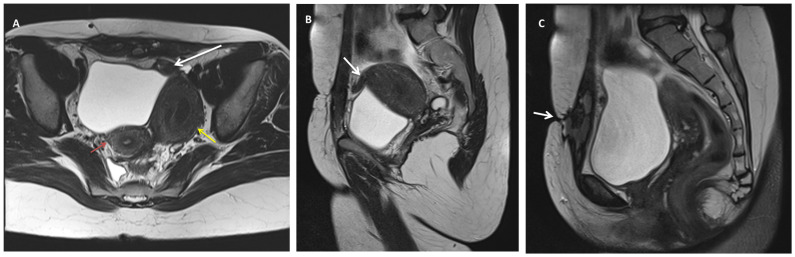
Pelvic MRI T2-weighted images depicting (A) axial view of a unicornuate right uterus (red arrow) and a large non-communicating cavitary left horn (U4a) with hematometra (yellow arrow). (B) sagittal view (white arrow) The white arrow is pointing to a fistulous trajectory towards anterior and medial directions. (C) that drains in the rectus abdominis muscles and subcutaneous tissue, reaching the scar tegument (arrow).
